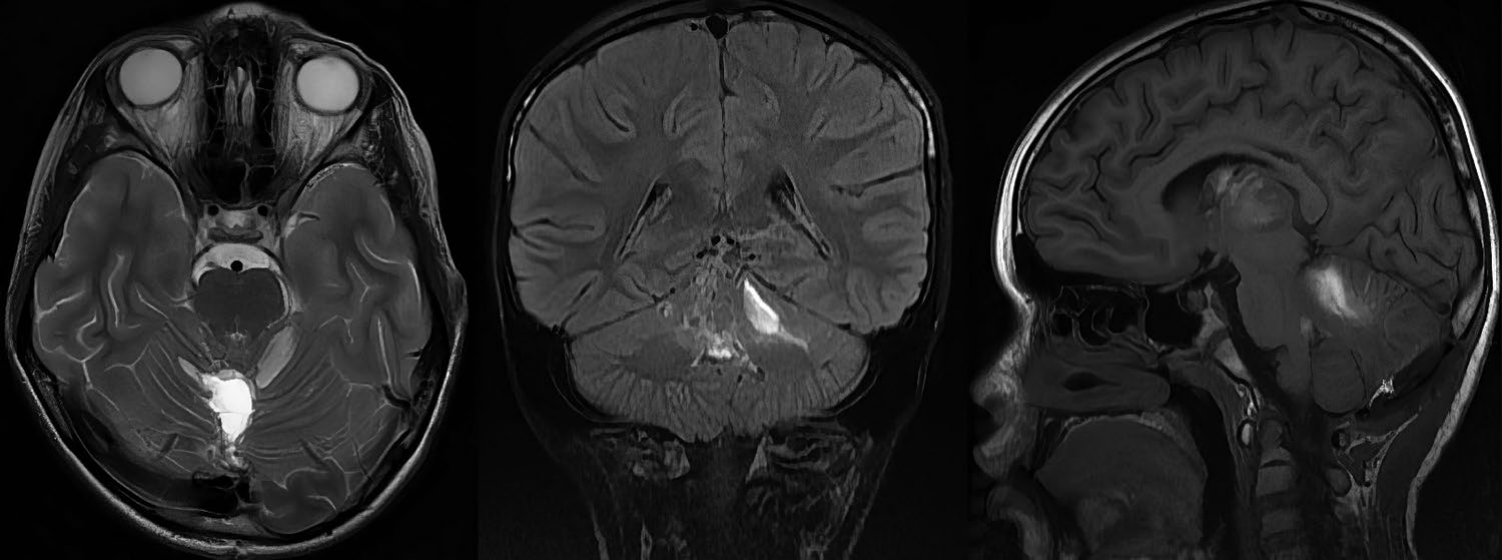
MRI findings from day 11
